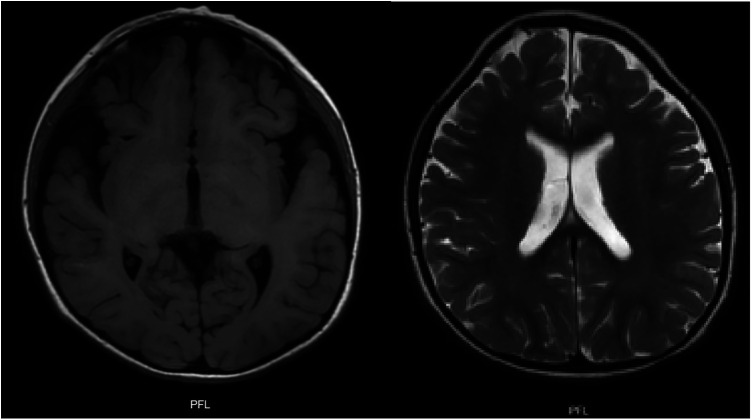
T1 and T2 weighted brain MRI: plagiocephaly, cortical atrophy, involvement of the white matter and frontal lobes is observed. Generalized increase in the amplitude of the subarachnoid space and ventricular system.
